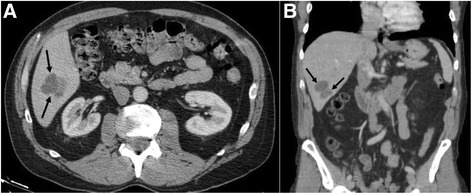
a Axial and b coronal post-contrast CT images through the abdomen before the start of treatment with evofosfamide demonstrates an index lobulated hypodense lesion (*arrows*) within the right hepatic lobe consistent with metastatic disease
